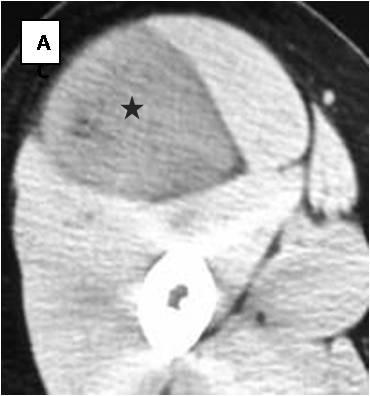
Unenhanced CT scan (1): A well-defined mass of attenuation close to muscle is located intermuscularly at the anterior aspect of the right upper thigh (asterisk).
